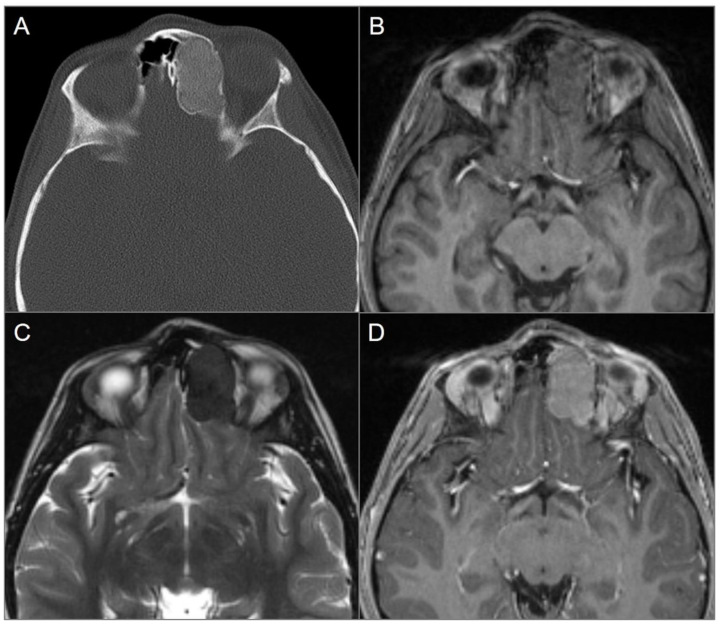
Ossifying fibroma. (A) Axial CT image shows an expansile and well-defined ground-glass density lesion with a thin sclerotic rim, centered in the left frontoethmoid sinuses. Note remodeling/scalloping of the left medial orbital wall and bulging into the left anterior cranial fossa. (B) T1W and (C) T2W axial images reveal isointense signal on T1 and markedly hypointense signal on T2. (D) Axial postcontrast T1W image demonstrates moderate homogenous enhancement of the lesion.
